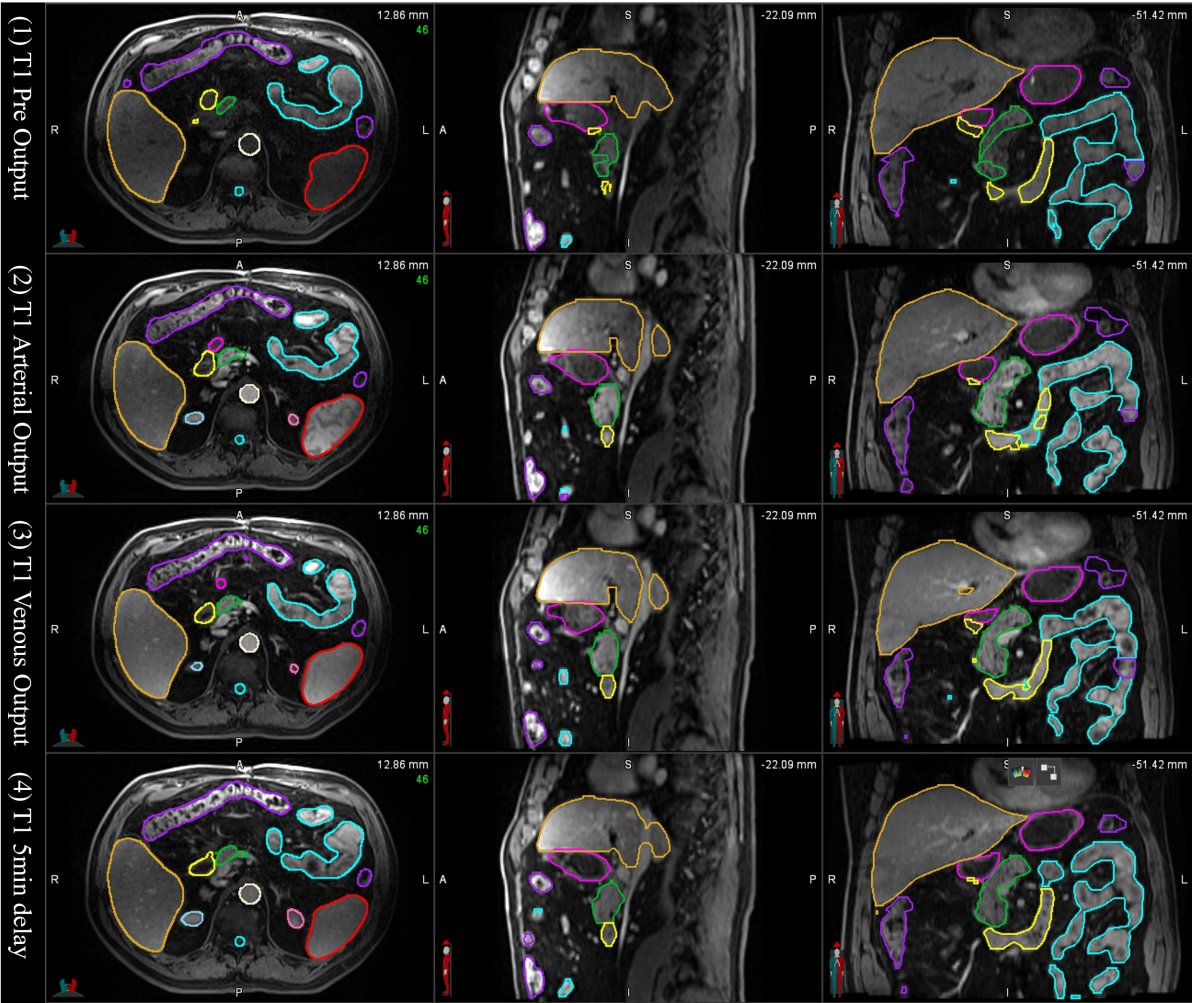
Auto-segmented contours by the multi-sequence mS-DLAS model on representative axial, sagittal and coronal images of 4 T1 weighted MRI sequences not used in the model training.
